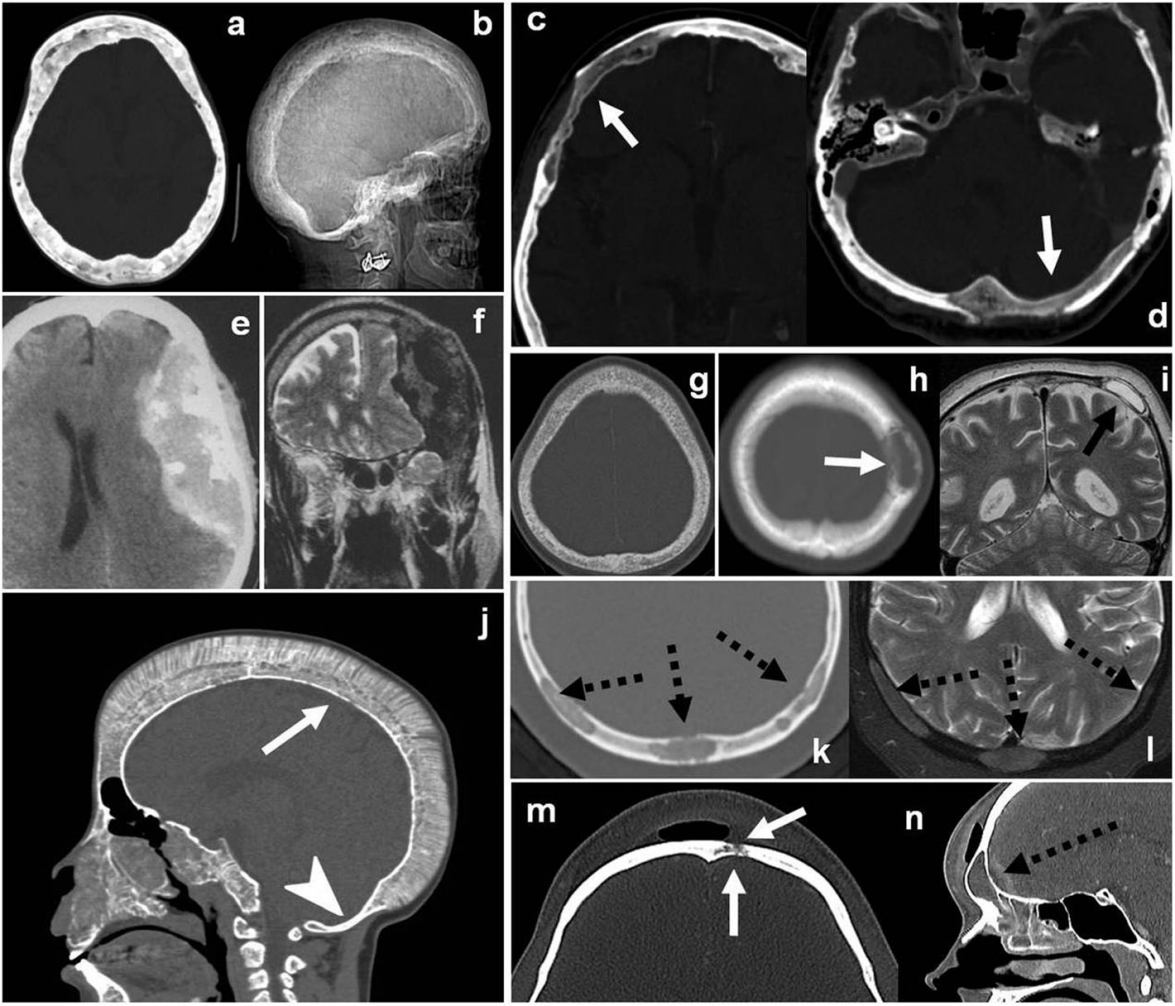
Miscellany. Paget disease (a, b) CT (a) and X-ray (b): Characteristic mixed bone lysis and sclerosis, cortical bone thickening, and expansion. Osteoporosis circumscripta cranii CT (c, d): large geographic radiolucent areas involving medullar and cortical bone in frontal and occipital regions (arrows). Amyloidoma (e, f) CT (e) and T2WI (f): Giant heterogeneous mass with marked T2 hypointensity and calcifications. Renal osteodystrophy CT (g): shows characteristic “salt-and-pepper pattern.” Brown tumor (h, i): unspecific well-defined, cystic appearance (arrows). Thalassemia CT (j): Diffuse diploic widening and “hair-on-end” appearance (arrow) with characteristic occipital bone preservation (arrowhead). Bone sarcoidosis (k, l) CT (k) and T2WI (l): Mixed predominantly lytic multiple lesions with lace-like internal pattern of calcification and T2 hypointensity (dashed arrows). Osteitis CT (m, n): Bone focal lysis and erosions of osteitis (arrows) contiguous to a frontal sinusitis complicated with intracranial laminar abscess (dashed arrow)
